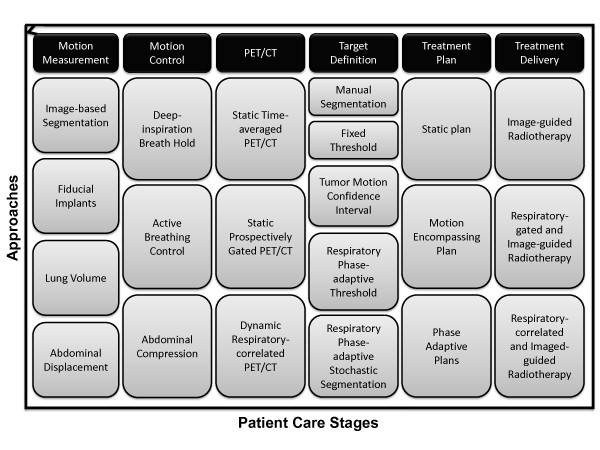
Landscape of potential approaches to patient care in motion-managed and PET/CT-guided radiotherapy of lung cancer. Across the stages of patient care, numerous approaches offer increasingly complex strategies. Details of each approach are given in Table
